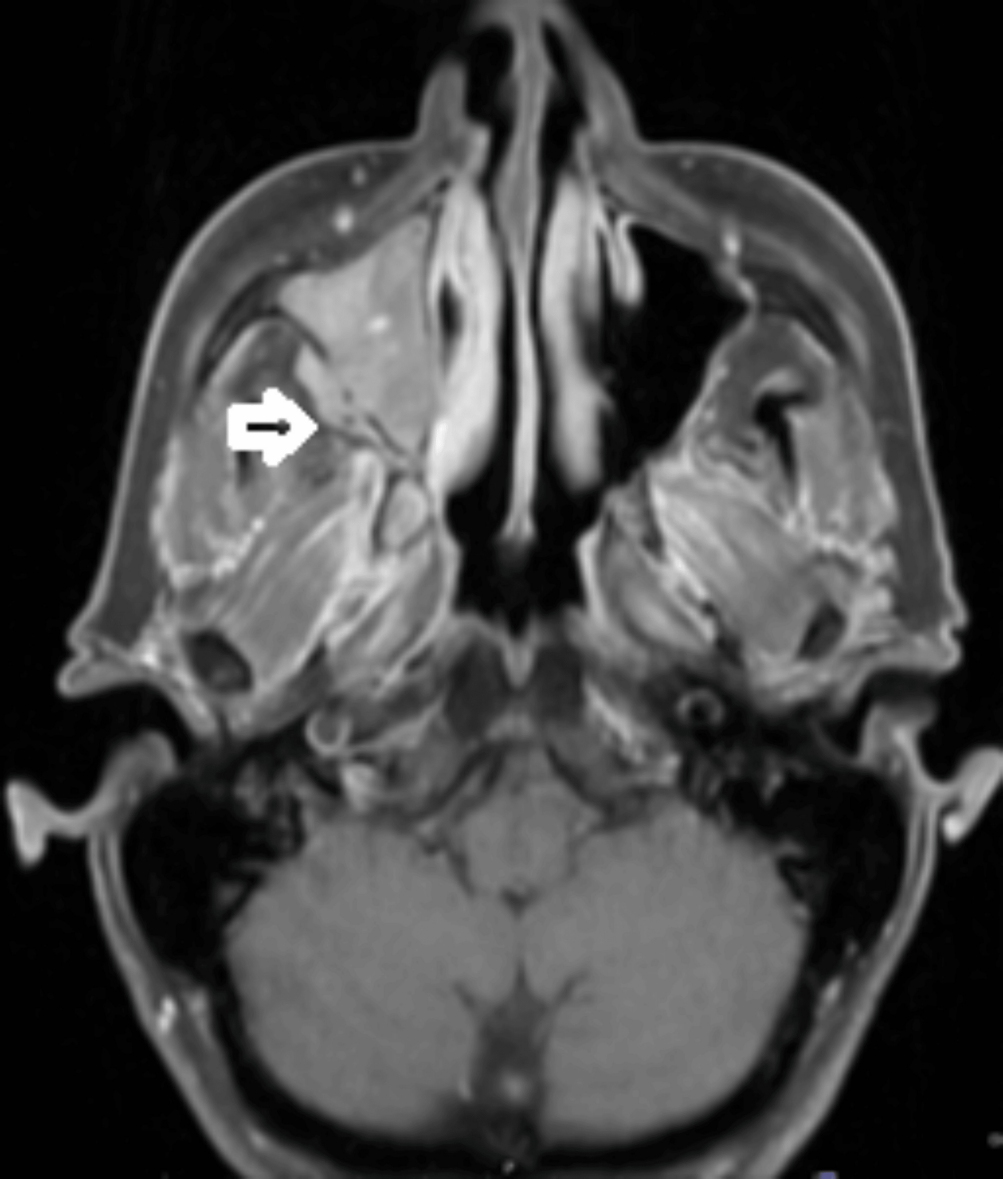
Post-gadolinium T1 weighted MRI Head in June 2022 (axial view) Slight enlargement of the intramaxillary recurrent tumour, which is also extending to the retromaxillary fat.
